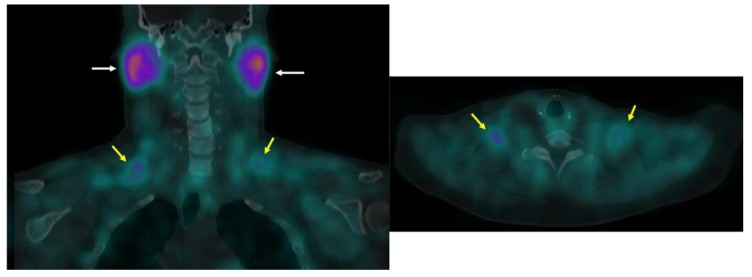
^99m^Tc-MIBI uptake in iBAT on a clinical scan. Supraclavicular BAT uptake shown coronal and axial fused ^99m^Tc-MIBI-SPECT/CT (yellow arrows). Salivary gland uptake (white arrows) is normal.
